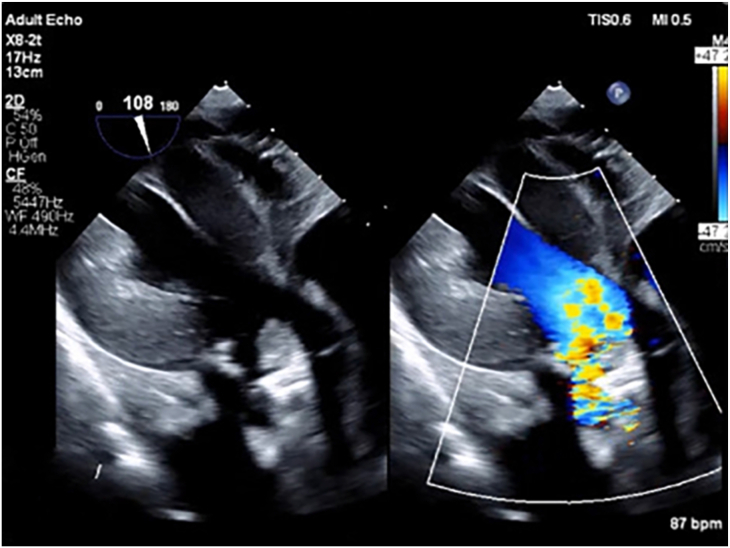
Transesophageal echocardiography image, transgastric long-axis view at 108 degrees (systolic frame), showing a large mass (vegetation) attached to the bileaflet mechanical aortic valve. In real time, color flow imaging revealed a significant paravalvular leak.
